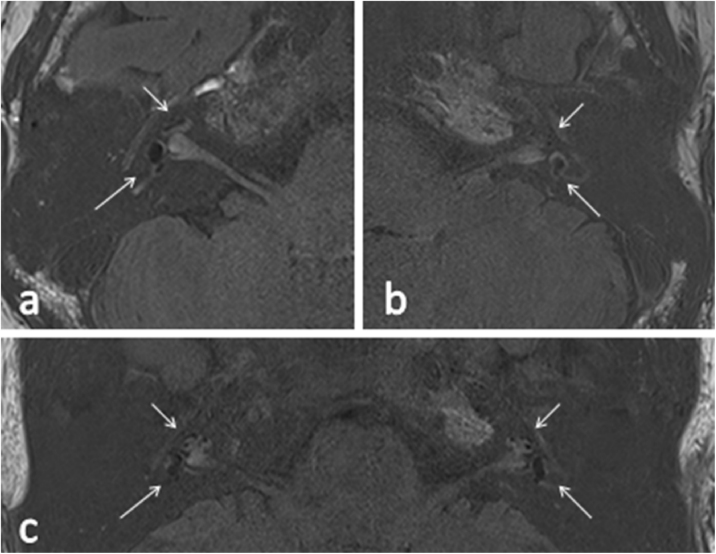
(a) A 53-year-old female with right Meniere’s disease, 3D-real IR MRI was performed after intravenous gadolinium injection in double doses. The long arrow indicated significant vestibular endolymphatic hydrops existed, and the short arrow indicated significant cochlear endolymphatic hydrops existed. (b) A 33-year-old male with left Meniere’s disease, 3D-real IR MRI was performed after intravenous gadolinium injection in double doses. The long arrow indicated mild vestibular endolymphatic hydrops existed, and the short arrow indicated mild cochlear endolymphatic hydrops existed. (c) A 29-year-old male with bilateral Meniere’s disease, 3D-real IR MRI was performed after intravenous gadolinium injection in double doses. The long arrow indicated significant vestibular endolymphatic hydrops existed, and the short arrow indicated significant cochlear endolymphatic hydrops existed.
